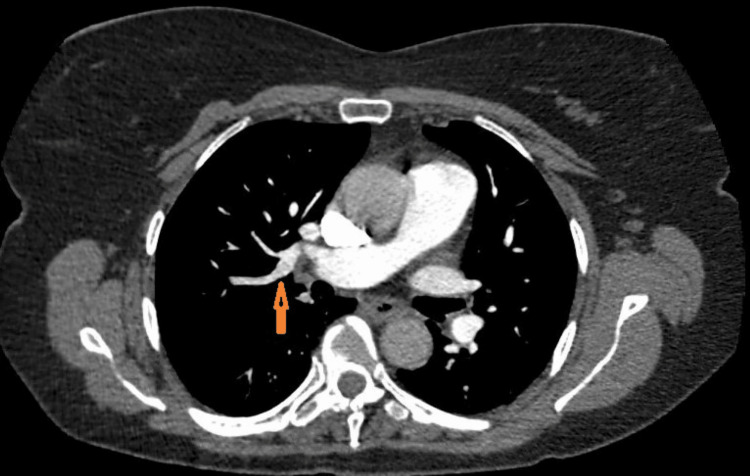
Pulmonary embolus seen on chest CT scan (arrow) CT: computed tomography
